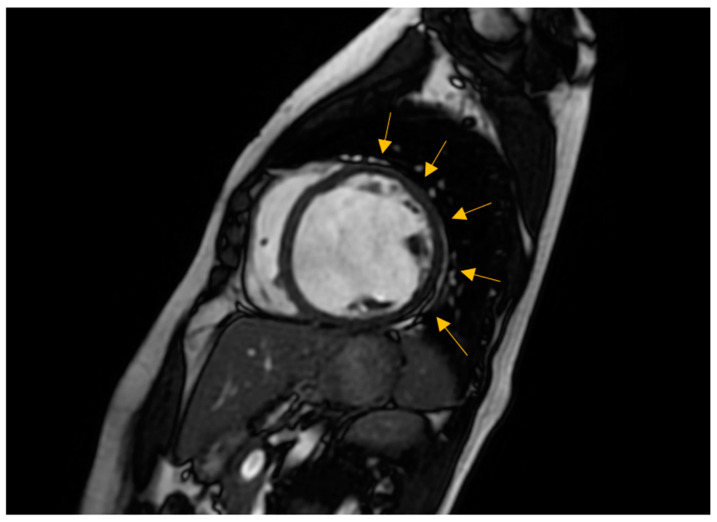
Cardiac magnetic resonance image, short axis. Dilated left ventricle with thin walls; late gadolinium enhancement highlights circumferential “ring-shape” mid wall fibrosis suggestive of desmoplakin cardiomyopathy (arrows).
